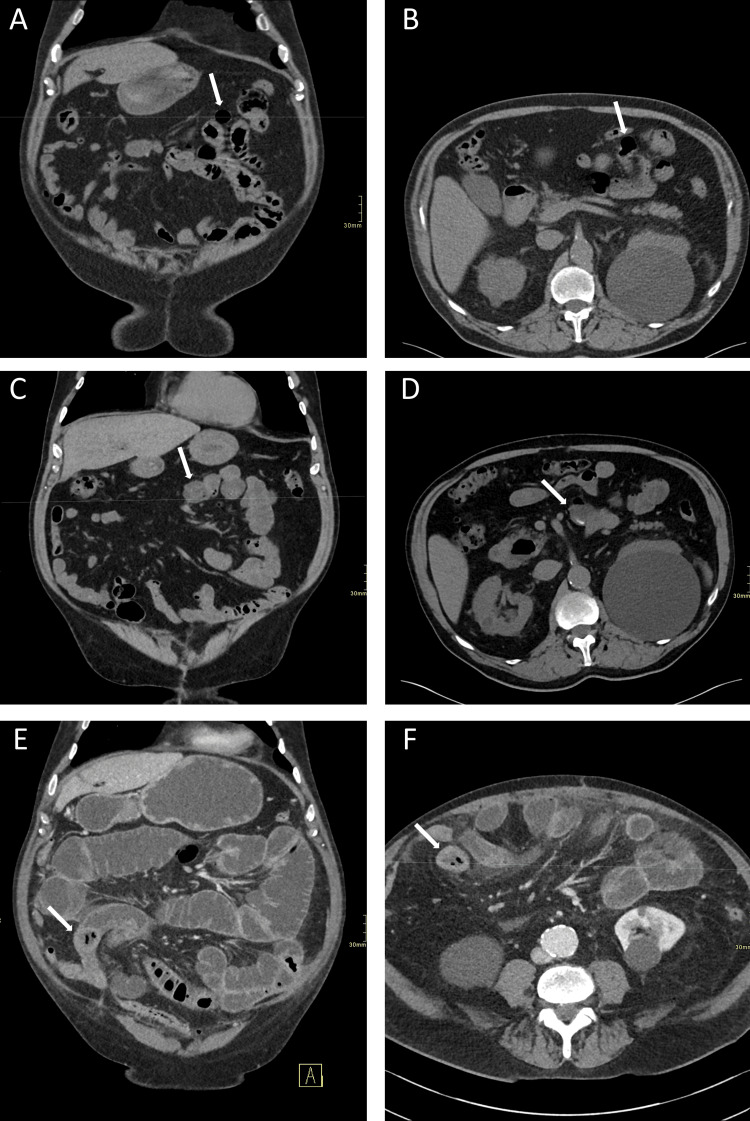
Evolution of the enterolith over seven years Non-contrast enhanced coronal (A) and axial (B) CT scan performed seven years prior to the current presentation showing an empty jejunal diverticulum (arrows). Non-contrast enhanced coronal (C) and axial (D) CT scan performed five years prior to the current presentation showing a jejunal diverticulum containing a partially hyperdense enterolith (arrows). Contrast-enhanced coronal (E) and axial (F) CT scan performed at the current presentation at the emergency room showing the previously seen enterolith, with a slightly hyperdense exterior and air within it, which has migrated and become impacted in the ilium (arrows), causing a proximal occlusion.
